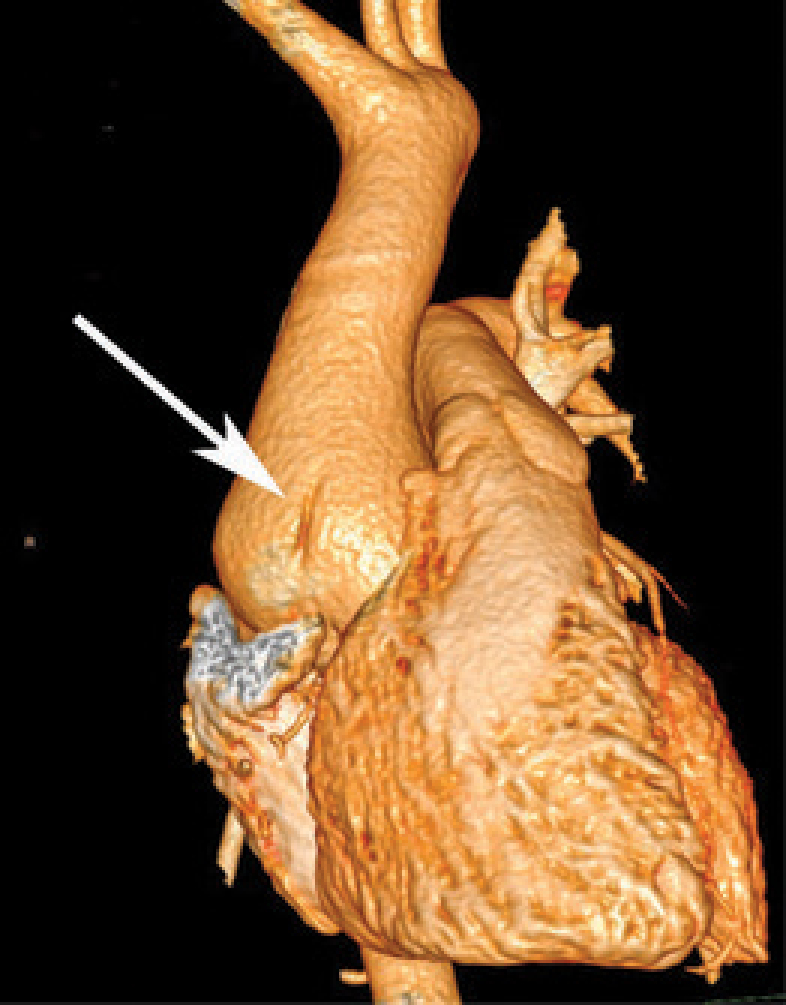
Volume rendering of an aortic CTA showing bulging of the wall.
